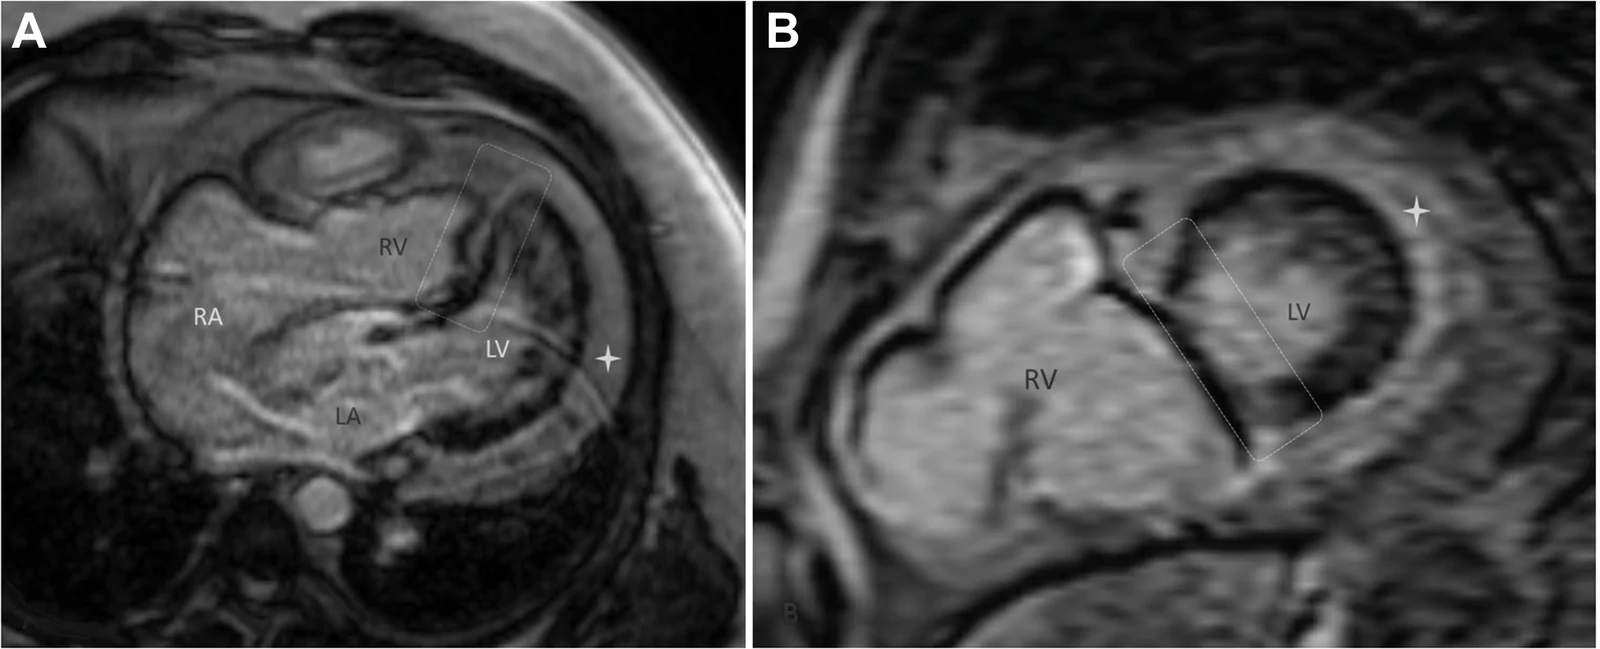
Cardiac magnetic resonance, late gadolinium enhancement. T1-weighted image, late gadolinium enhancement (LGE). A: axial view; B: short axis, two-chamber view. Late intramyocardial septal enhancement is observed in the basal inferoseptal (segment 3), mid inferoseptal (segment 10), and mid inferior (segment 11). No late enhancement was evident in the right ventricle. ✦: Lipomatosis of epicardial fat.
